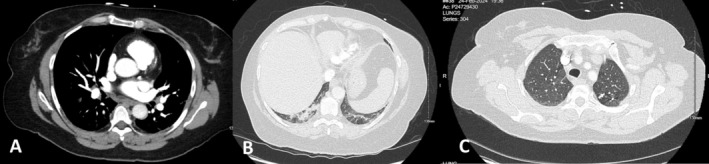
Computed Tomography Angiography visualizing bilateral pulmonary embolisms (A), right lower lobe pulmonary infarct (B), and a left upper lobe pulmonary arteriovenous malformations status post coiling (C).
